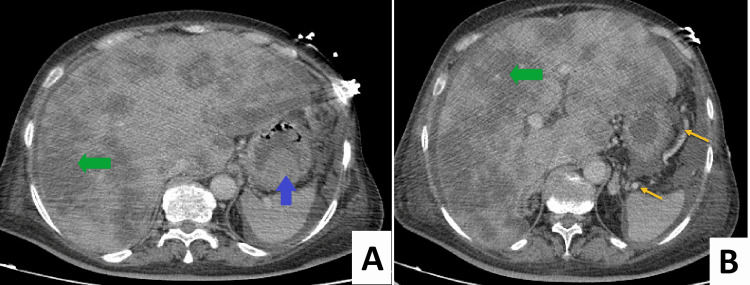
Axial CT scan Axial CT scan shows multiple liver metastases (green arrows in A and B), gastric blood (blue arrow in A), and perigastric collateral veins (yellow arrows in B)
